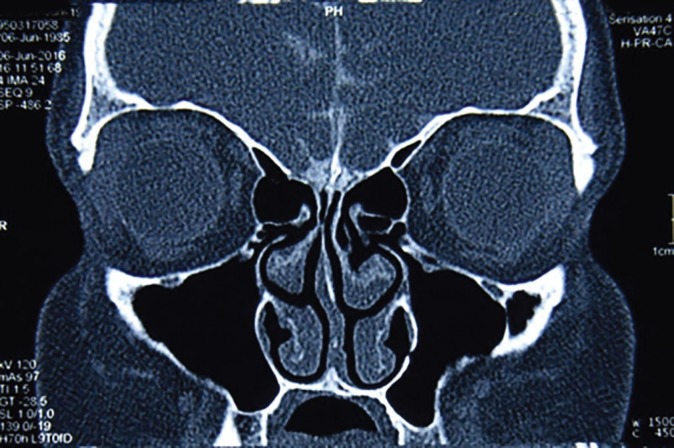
Postoperative coronal CT of th nasal area. Caudal septal relocation and bilateral open airway are evident.
